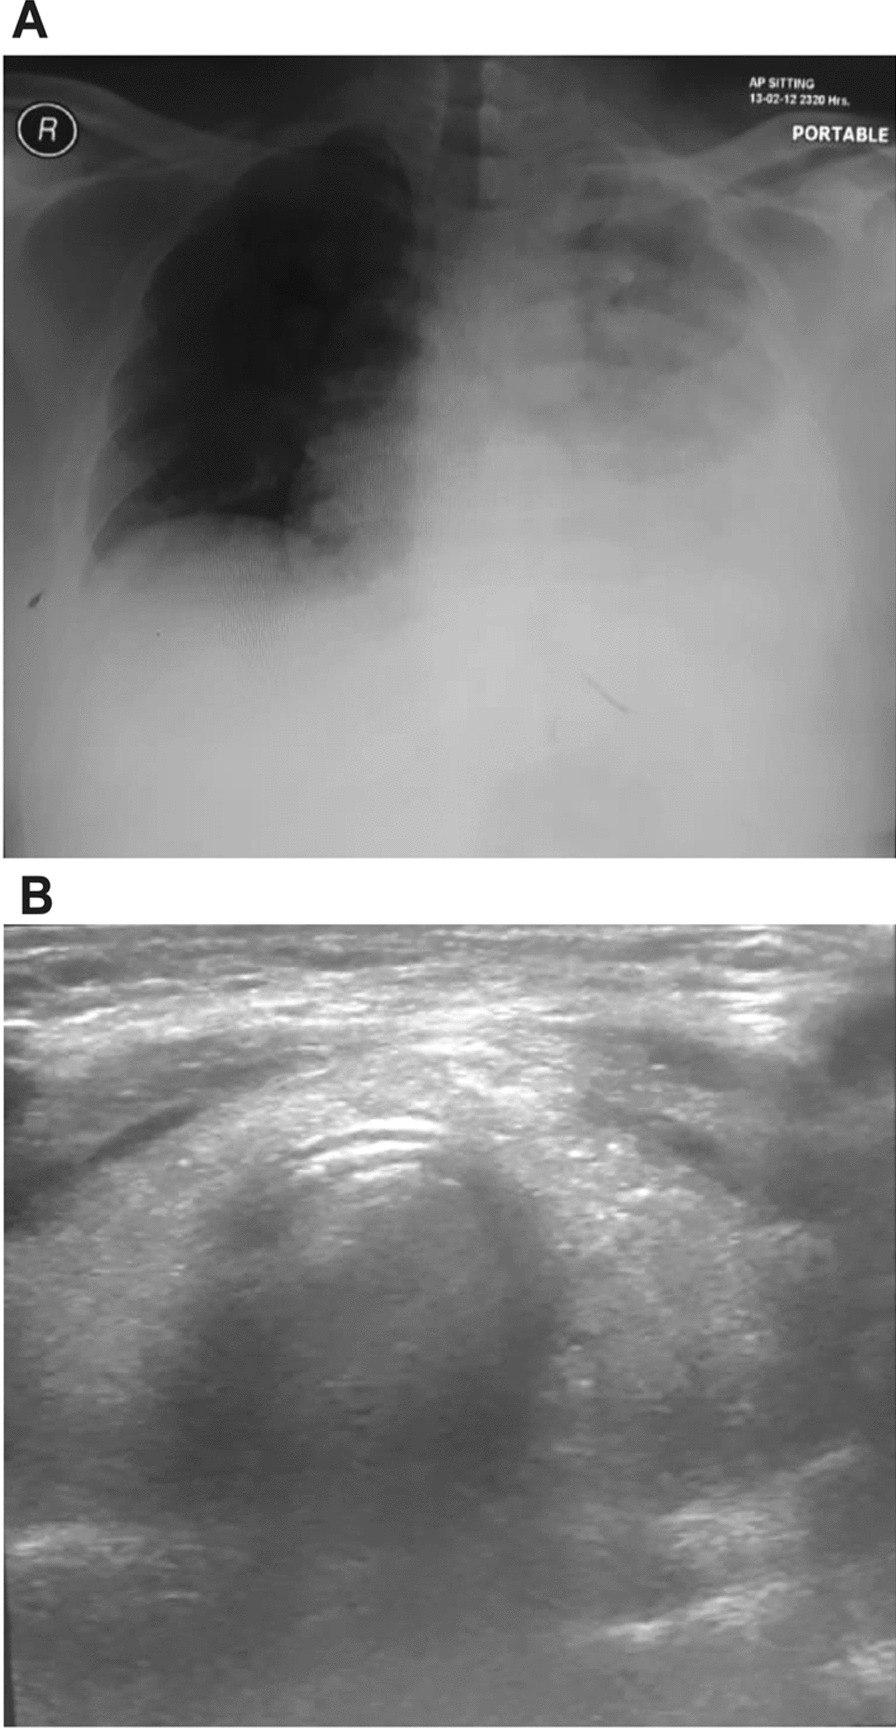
X-ray and ultrasonography of the chest: chest X-ray showing left side pleural effusion with mediastinal shifting to the right, the right lung is clear (A).B Ultrasonography of the lungs shows a left-sided collection of pleural effusion presenting as a crescent-shaped hypoechoic area measured as 8 × 1.9 cm
